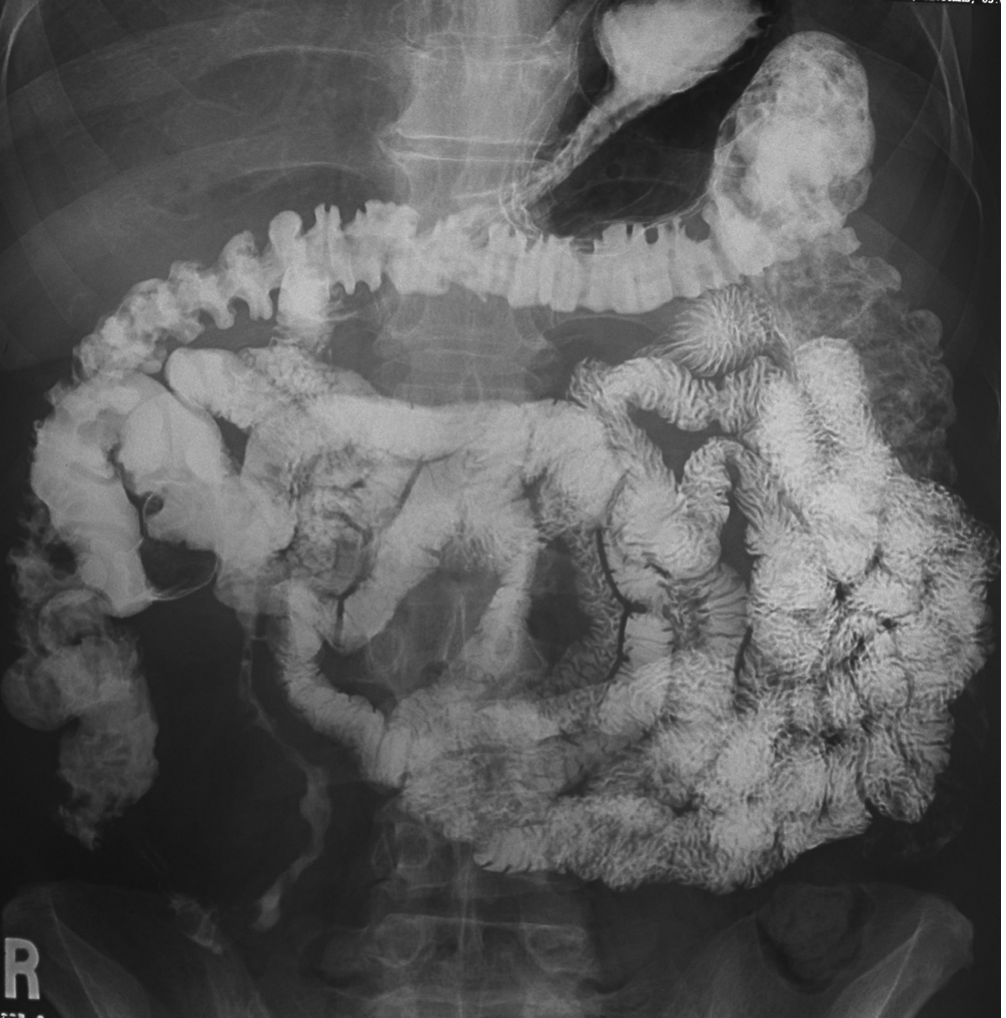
X-ray demonstrating 18 cm of abnormal small bowel in the distal ileum with effacement of the small bowel folds.
